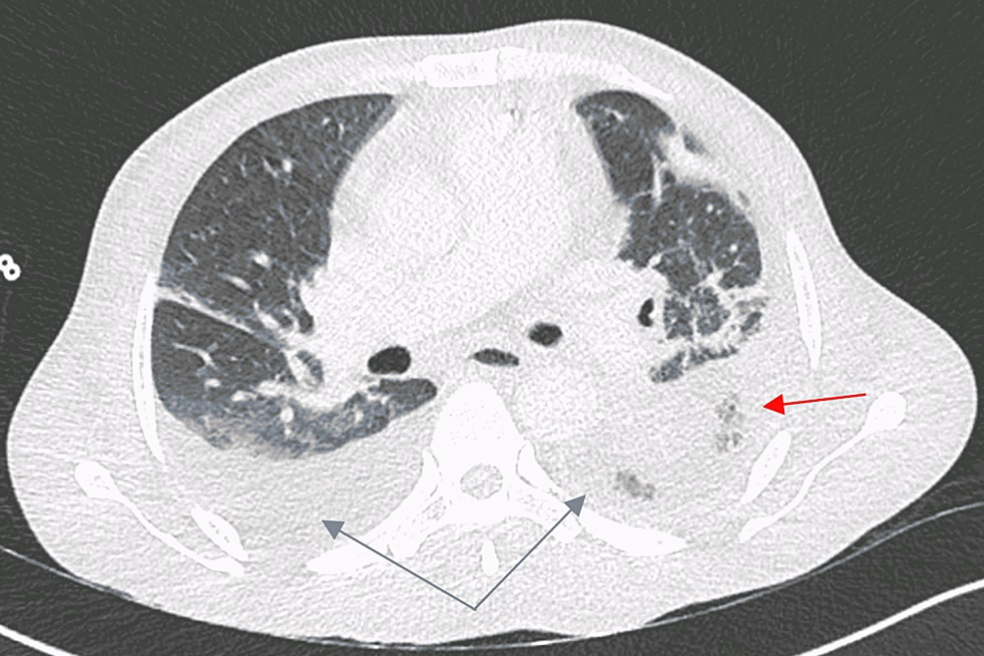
Thorax CT scan showing bilateral pleural effusion and parenchymal densification of the left lung Gray arrows: bilateral pleural effusion; red arrow: parenchymal densification of the left lung.
